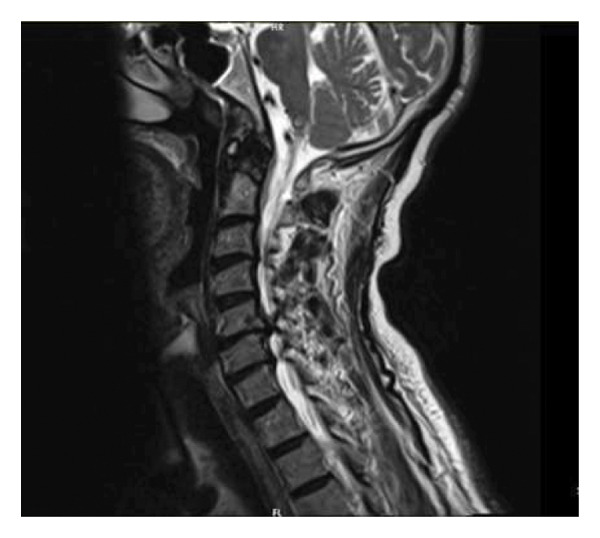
Preoperative T2‐W MRI: severe canal stenosis is seen at C5/C6 level and a disc bulge is causing mild canal stenosis and moderate bilateral neural foramen stenosis at C6/C7 level.
